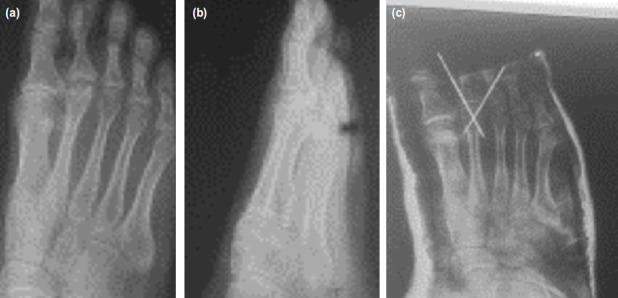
The radiographs show osteotomy where no debridement was done; (a) anteroposterior view, (b) lateral view and (c) postoperative view.
